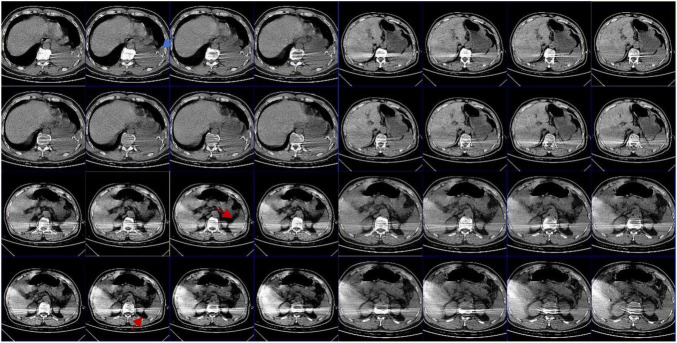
Abdominal CT: the blue arrow shows intrathoracic drain; the red arrow shows that there appears to be exudate around the spleen; the splenic hilum appears to be intact.
